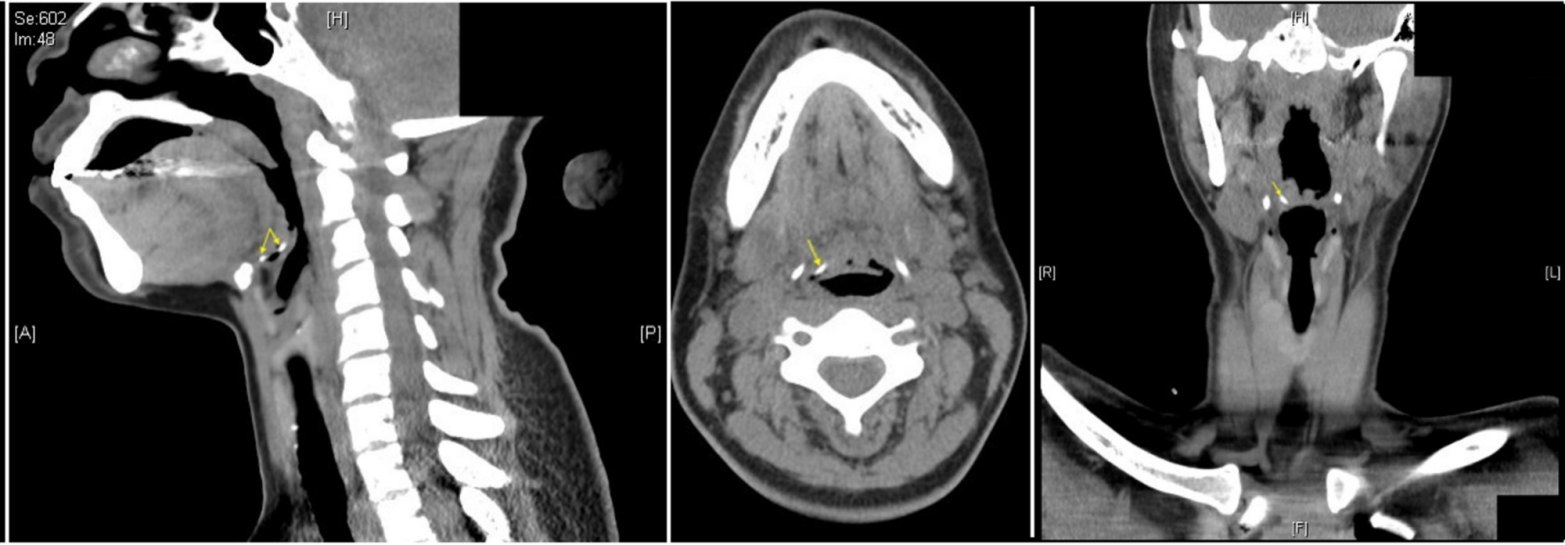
CT panel demonstrating the fish bone right vallecula (arrows).
